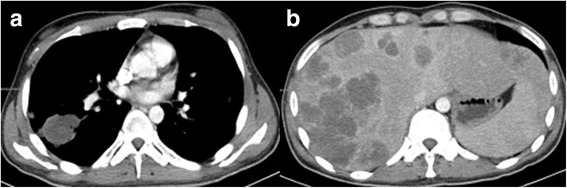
a (left): CT showing that the lung lesions present in Fig. 18a have increased in number and size despite ITP therapy. b (right): The liver lesions present in Fig. 18b have also increased in number and size
